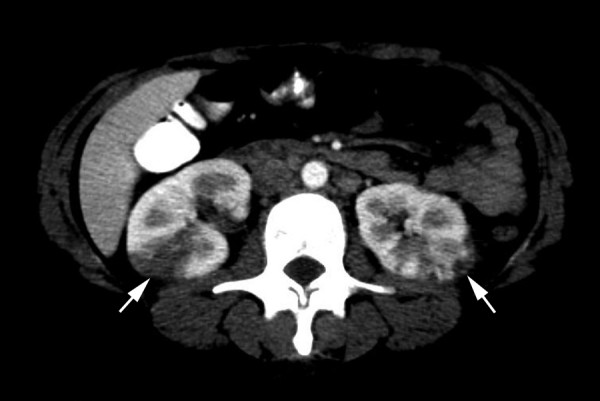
Abdominal computed tomography scan showing multiple wedge defects in both kidneys.
